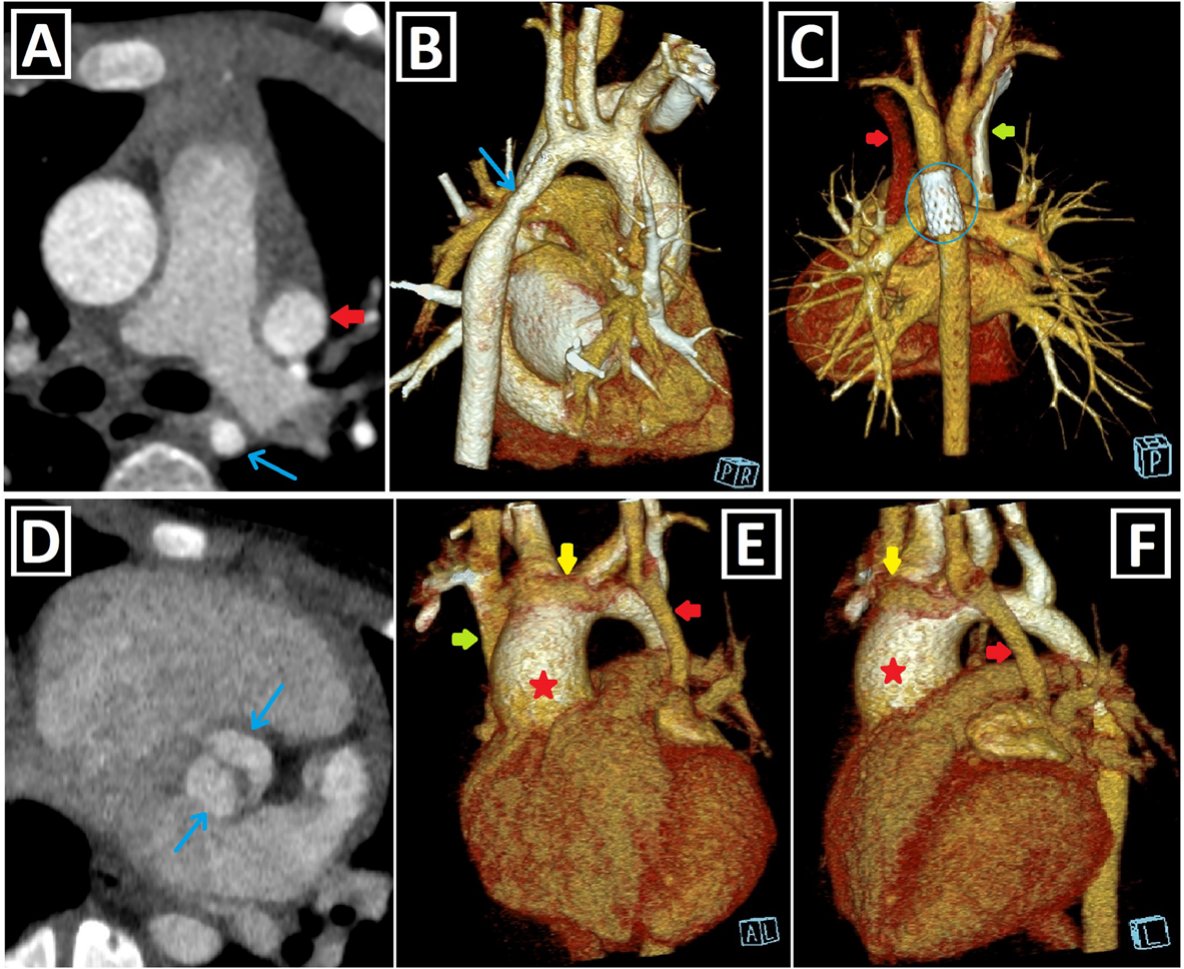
Left-sided obstructive lesions accompanying PLSVC. a–c Axial (a) and 3D VRT (b, c) CT images. The narrow segment compatible with aortic coarctation (blue arrows) is seen in a patient with PLSVC (red arrow) (a, b). Aortic coarctation treated by the endovascular intervention (blue circle) is depicted in another patient with double SVC (green and red arrows) (c). d–f Axial (d) and 3D VRT (e, f) CT images show the bicuspid aortic valve (blue arrows) (d) and the accompanying ascending aorta dilation (red stars) (e, f) in the patient with double SVC (green and red arrows). A bridging vein between RSVC and PLSVC (yellow arrows) is also seen (e, f)
